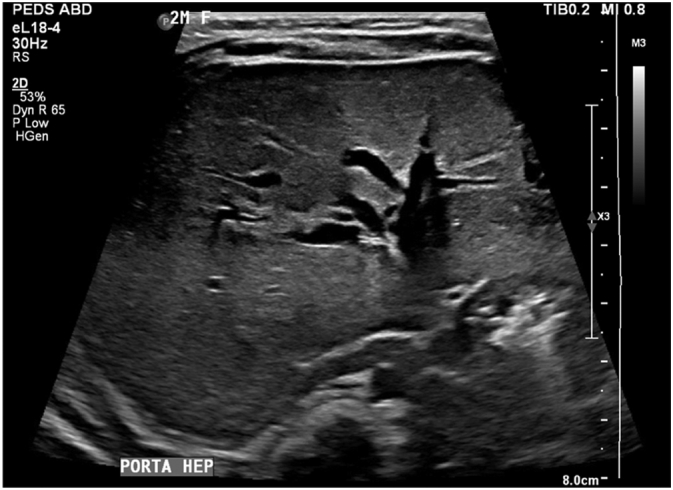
Ultrasound of liver at the porta hepatis showing complete absence of a gallbladder structure.
